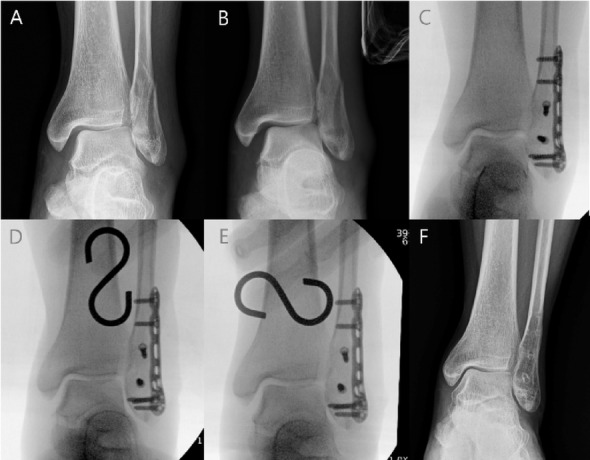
Representative radiographs of a 54-year-old woman with a lateral malleolar fracture. A Preoperative anteroposterior ankle radiograph. B Preoperative gravity stress radiograph. C Intraoperative fluoroscopic image after lateral malleolar fixation. D Intraoperative valgus stress view. E Intraoperative external rotation stress view. These stress views showed no medial instability after fixation; therefore, deltoid ligament repair was not performed. F Standing anteroposterior ankle radiograph obtained 14 months postoperatively
